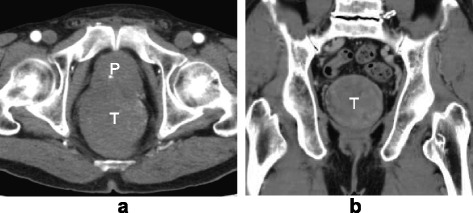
Contrast-enhanced computed tomography: a horizontal and b coronal sections. A large circle mass, 7.5 cm in diameter, was detected at the lower rectum. It occupied almost all space of the lesser pelvis. *P* prostate, *T* tumor
